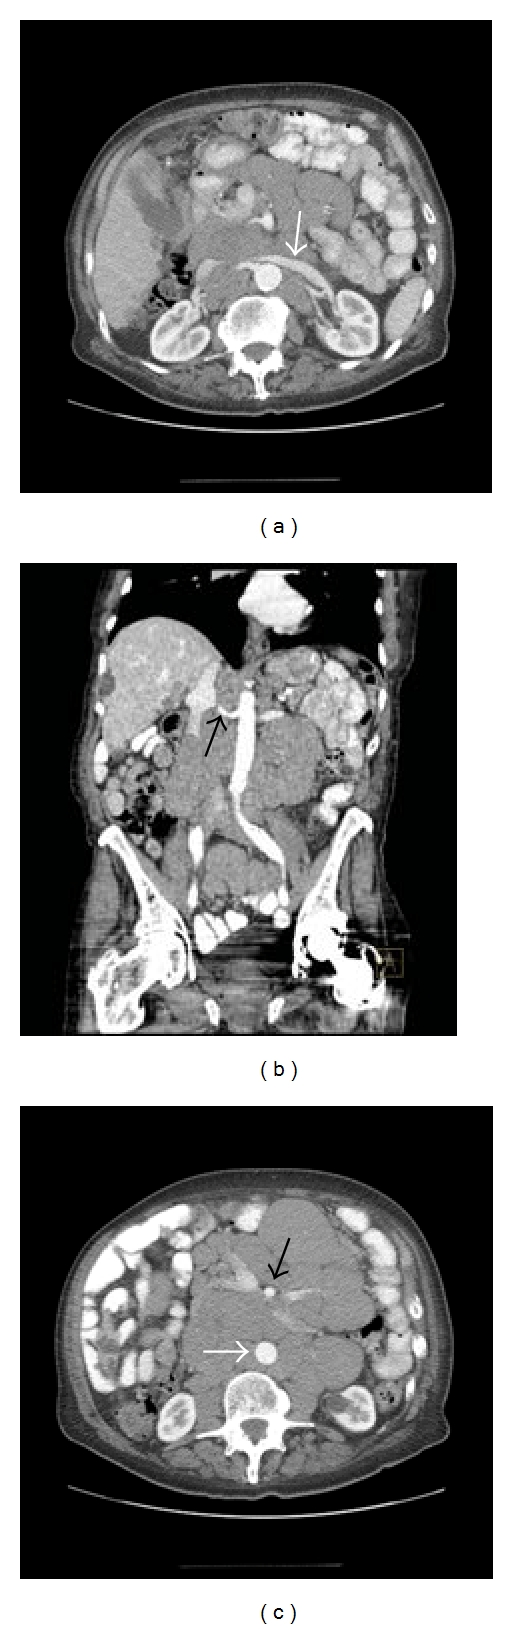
Axial (a) and coronal (b) contrast enhanced CT of the abdomen in a patient with chronic lymphocytic leukemia displaying encasement of vessels such as the left renal vein (white arrow) and right renal artery (black arrow) by lymph nodes masses, the so-called “sandwich sign.” Axial contrast enhanced CT (c) of the abdomen at a lower level in the same patient displaying elevation of the abdominal aorta off the vertebral column (white arrow) which is a characteristic feature of lymphoma and helps to differentiate lymphoma from other retroperitoneal masses, such as retroperitoneal fibrosis. Note the encasement of the superior mesenteric artery (SMA) by the lymph node mass and increased separation of the SMA from the aorta (black arrow).
